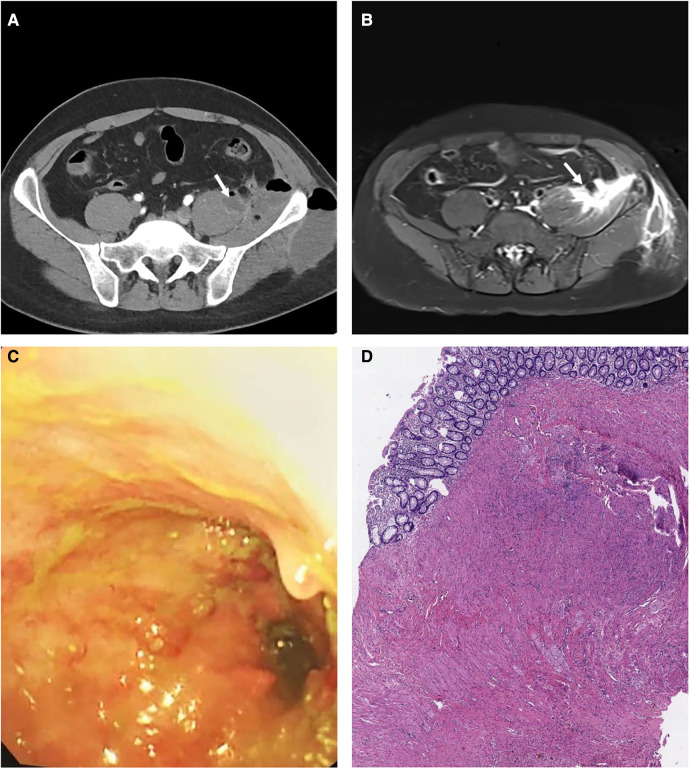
The abdominal CT showed patchy low-density shadow of the left pelvic wall soft tissue (A). The pelvic MRI showed that the lower part of the left psoas major muscle, the left pelvic wall and the left buttock were thickened with multiple high signal shadows (B). Colonoscopy showed multiple filling defects and local lumen stenosis in the terminal ileum, ileocecum and the beginning of the ascending colon (C). The pathological results revealed that a large number of proliferative ganglion cells and circuitous hyperplastic nerve fibers were found in the submucosa and muscular layer of the colon wall (D).
